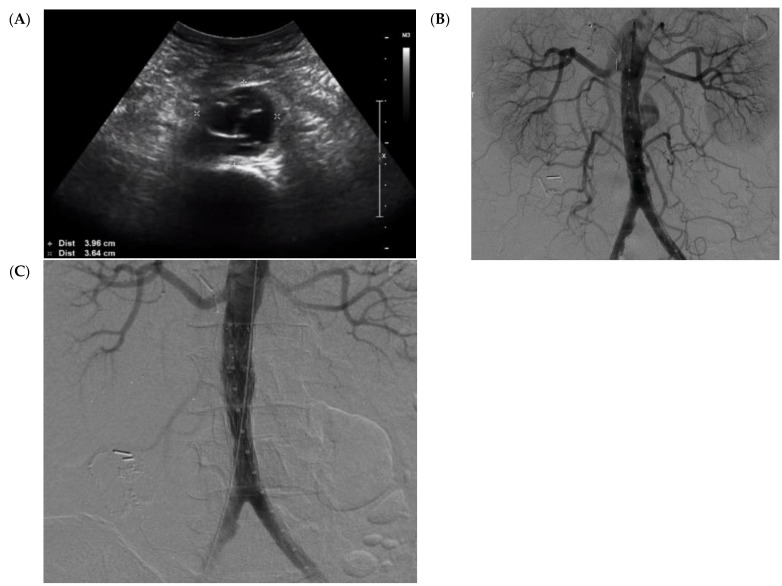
(A) Preoperative duplex ultrasound demonstrating rapid expansion of an infra-renal mycotic aortic aneurysm to a maximum diameter of 3.96 cm. (B) Anterior posterior intraoperative angiogram demonstrating a mycotic aneurysm. (C) Final intraoperative angiogram demonstrating a sealed mycotic aneurysm using an endovascular stent graft.
